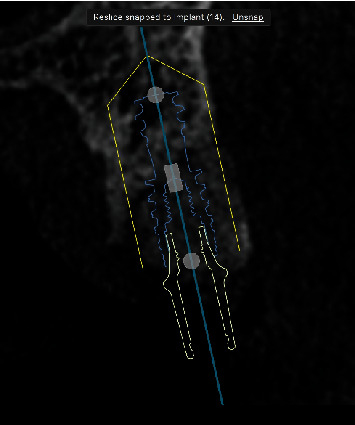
CBCT scan showing adequate bone volume to proceed with the implant placement.
